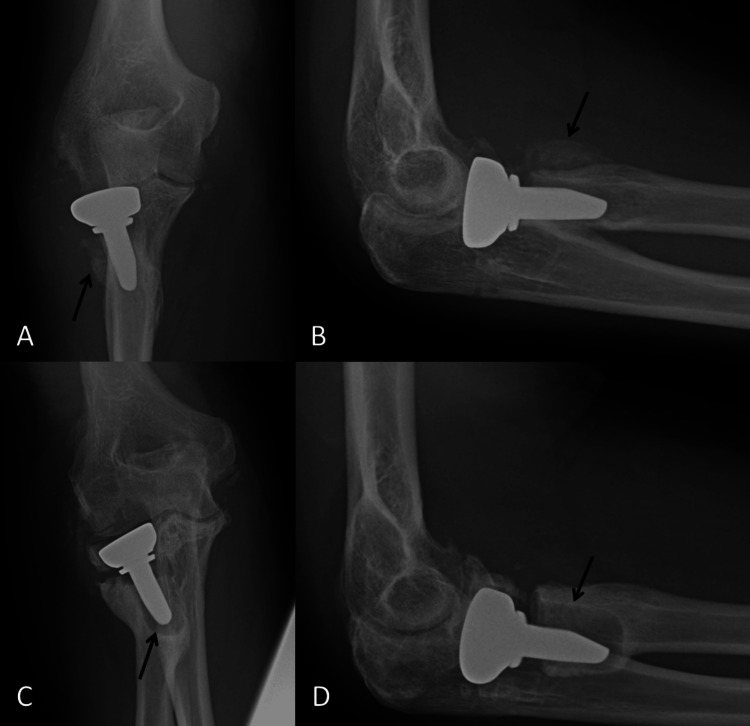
Post-operative radiographs at four weeks and 12 months after surgery. A: Antero-posterior view at four weeks after surgery demonstrating callus formation at the resection level of the radial neck (black arrow). B: Lateral view at four weeks after surgery demonstrating the same callus formation as image A (black arrow). C: Antero-posterior view at twelve months after surgery demonstrating significant loosening of the implant (black arrow). D: Lateral view at 12 months after surgery demonstrating significant loosening of the implant (black arrow).
